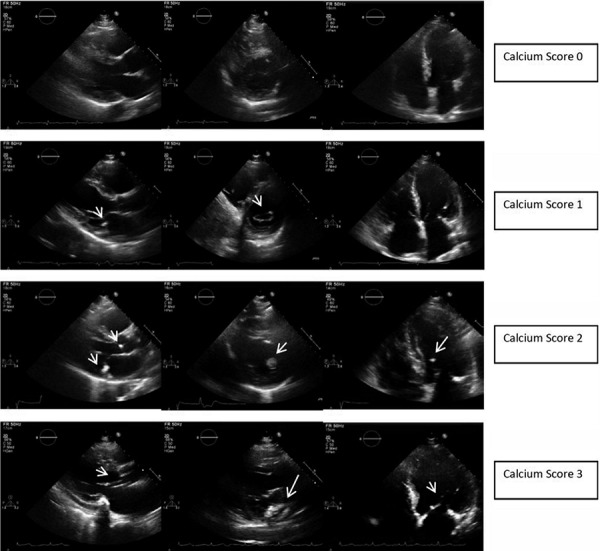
Echocardiographic cardiac valvular calcium scoring profile. Echocardiographic snapshots demonstrating the extent and severity of cardiac calcification, which was used to derive an overall calcification score. Cardiac calcification predominantly affected the mitral valvular annulus, leaflets, and chordae tendineae as well as the aortic valve leaflets and annulus.
